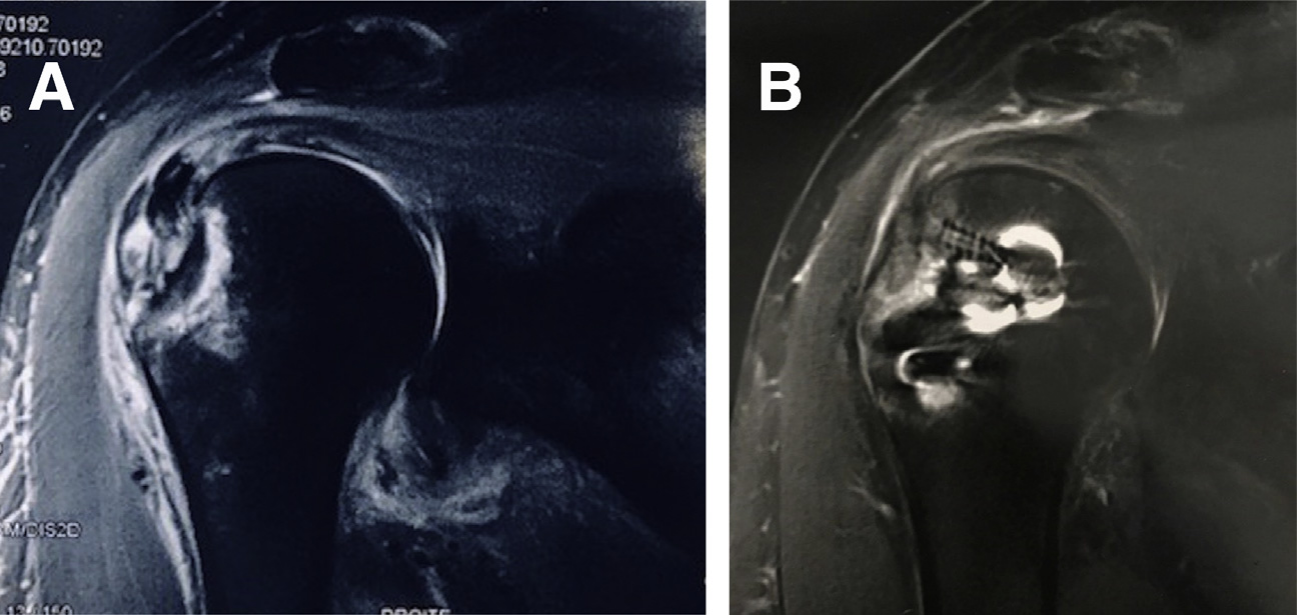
(A) Magnetic resonance imaging (MRI) frontal view of the right shoulder showing comminuted displaced fracture with the supraspinatus tendon. (B) At the last follow-up, MRI of the right shoulder shows healed cuff and greater tuberosity fracture.
